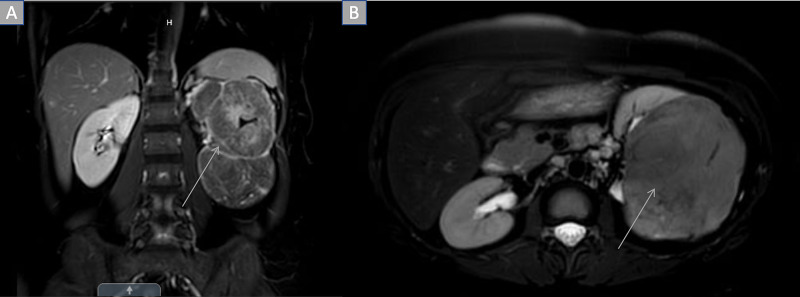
MRI abdomen demonstrating left renal mass A: Coronal view of exophytic left renal mass; B: Transverse view of left renal mass.
